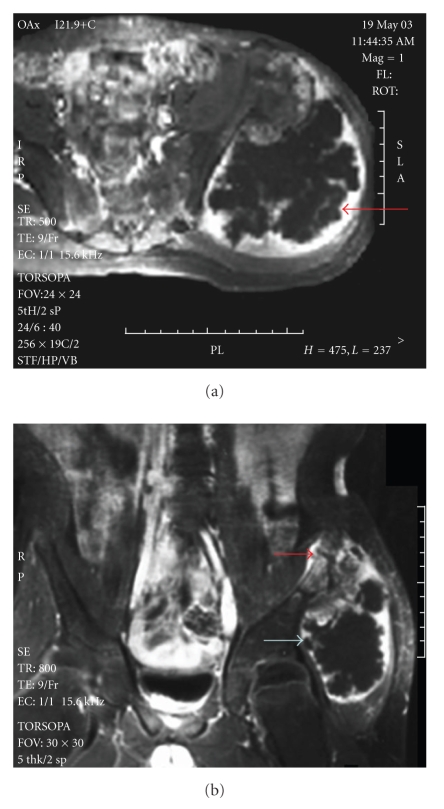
(a) Axial MRI images of the iliac mass reveal its enlarged cartilage cap of 5-6 cm thickness and the nodular or ring and arc enhancement (large arrow). (b) Coronal MRI images reveal the enlarged cartilage cap, nodular or ring and arc enhancement (large arrow), and invasion of the inner table cortex by tumor (small arrow).
